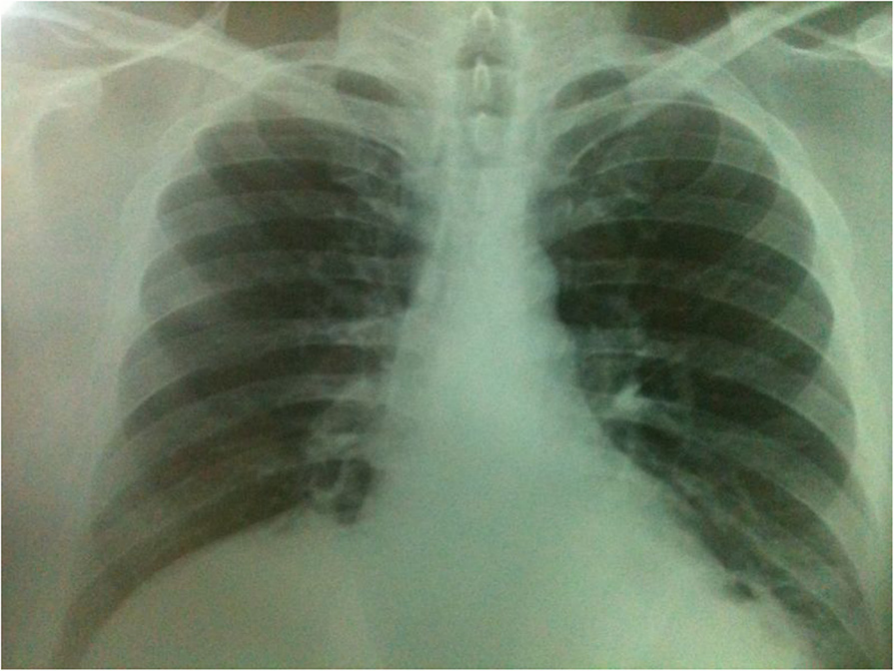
Postoperative X-ray taken the day after the procedure.
